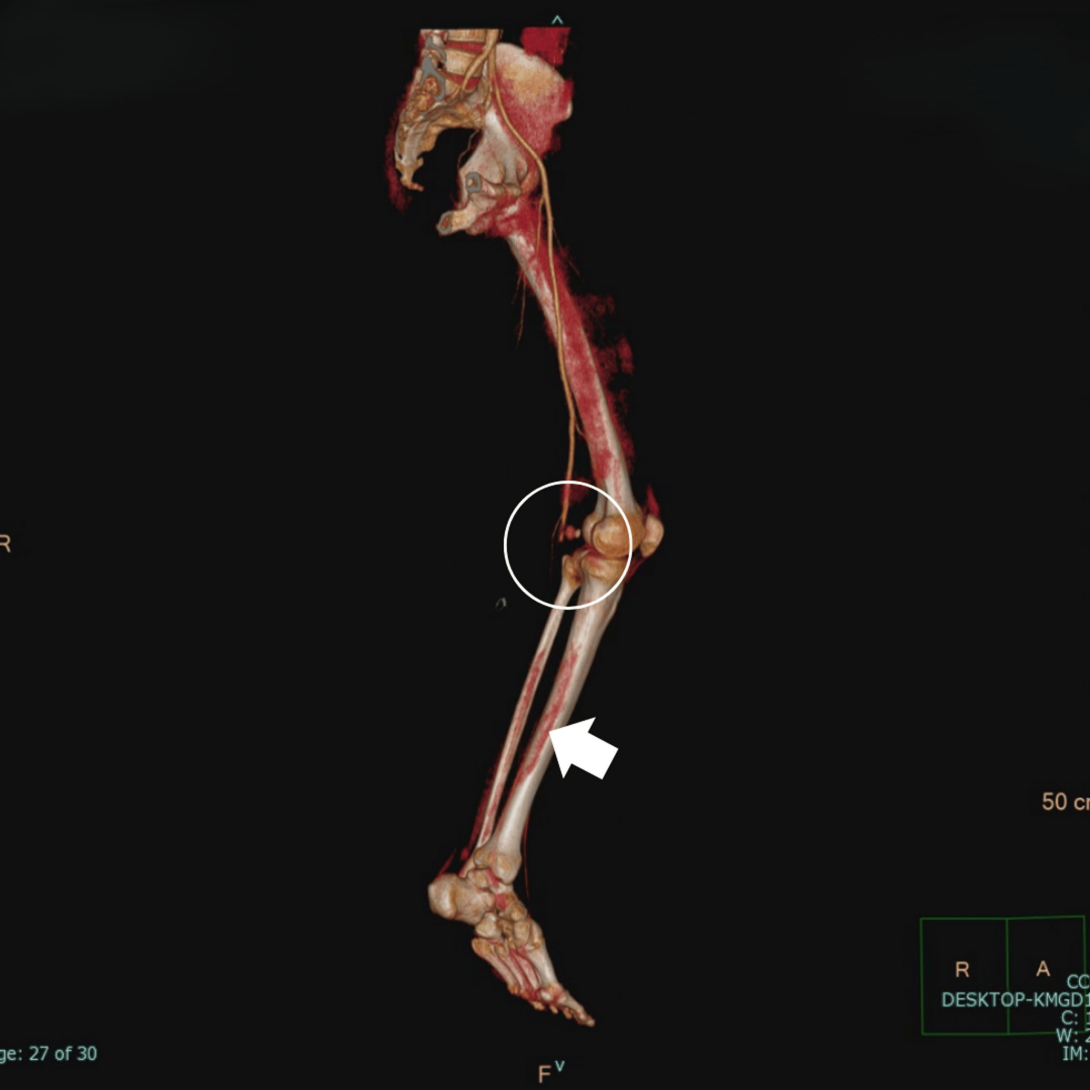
CT angiography showing distal popliteal artery injury with collateral perfusion CT angiography with vascular reconstruction demonstrated vascular compromise with pseudoaneurysm formation at the P2 segment (retroarticular), extending into the P3 segment (proximal tibial), resulting in an absence of distal flow in the popliteal artery, findings suggestive of vascular injury at this level (white circle). The tibioperoneal trunk was not visualized; however, faint contrast enhancement was observed in the distal vascular beds, indicating limited perfusion likely sustained via collateral arterial pathways (white arrow). CT: computed tomography
